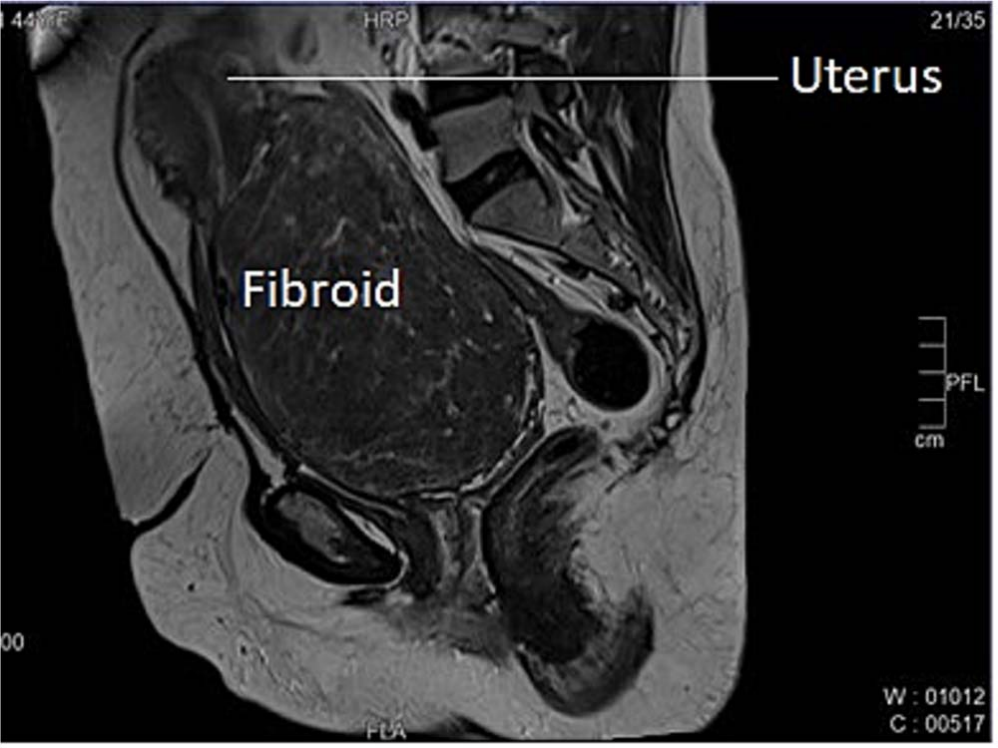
Longitudinal section of MRI image of Case 1 – shows uterus sitting on the top of large cervical fibroid, giving the appearance of a lantern on saint Paul’s dome.
